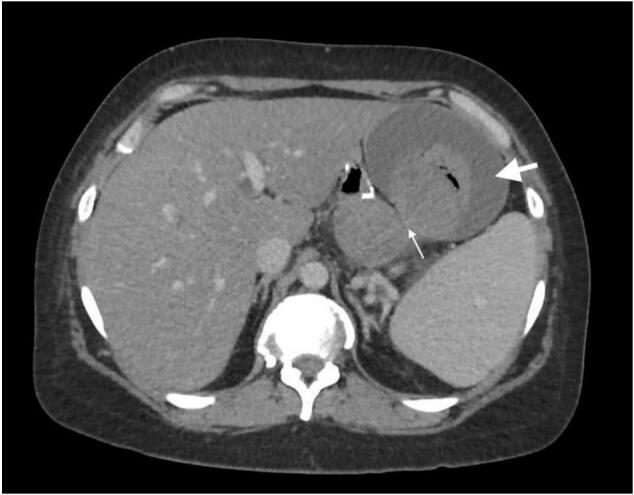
Initial CT scan. There is a fistulous communication between the excluded stomach (small arrow) and blood dissecting the wall components of the remnant stomach (big arrow).
